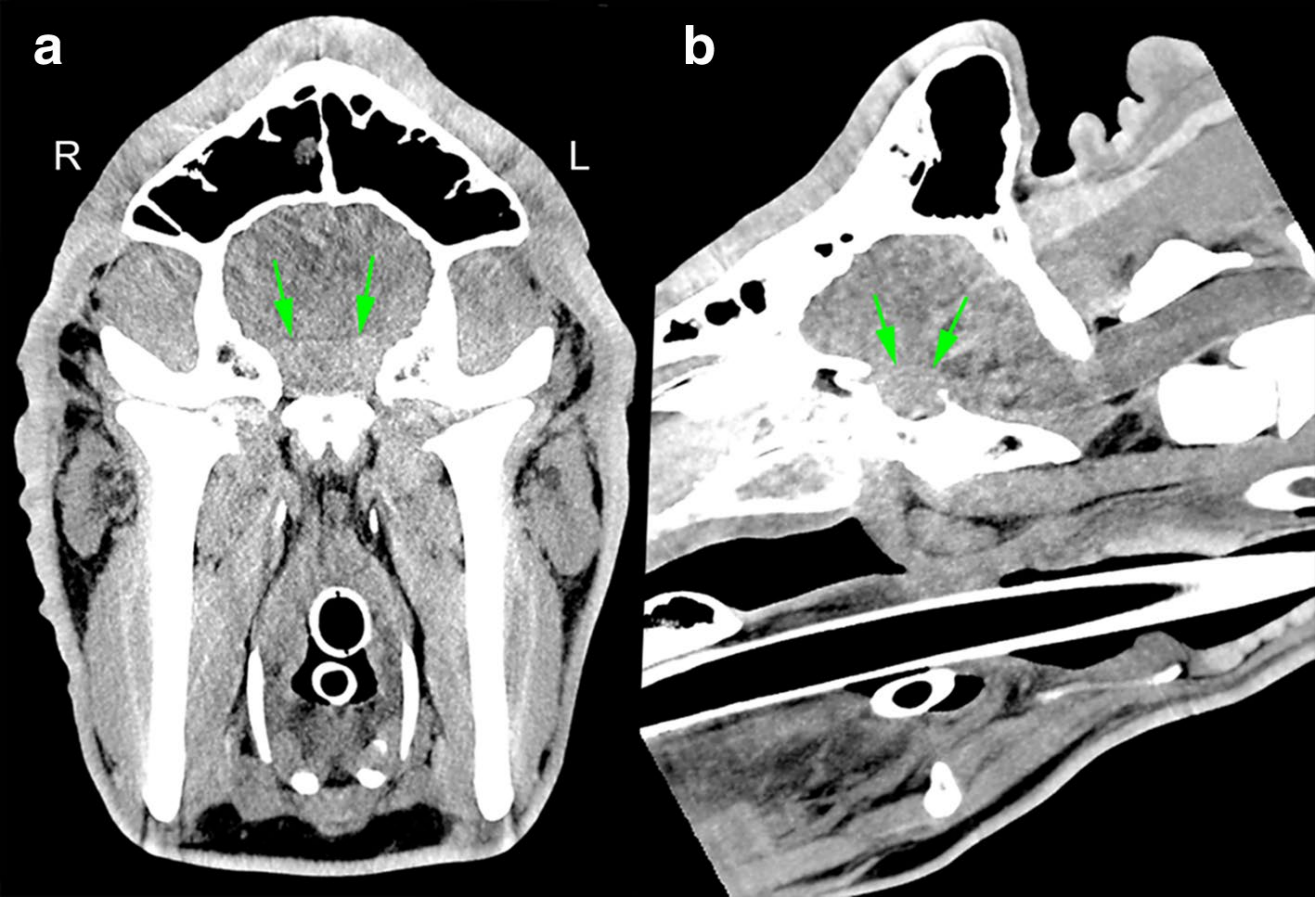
Pre-contrast CT image. Transverse a and sagittal b pre-contrast CT image reconstruction in a soft tissue algorithm (window level 50, window width 200) of the brain of a Holstein–Friesian bull with abscess of the hypophysis. The hypophysis (*green arrows*) had a heterogeneous attenuation pattern and a higher attenuation value than the surrounding brain parenchyma. The attenuation value was slightly lower on the *left side* of the hypophysis than on the *right side*
